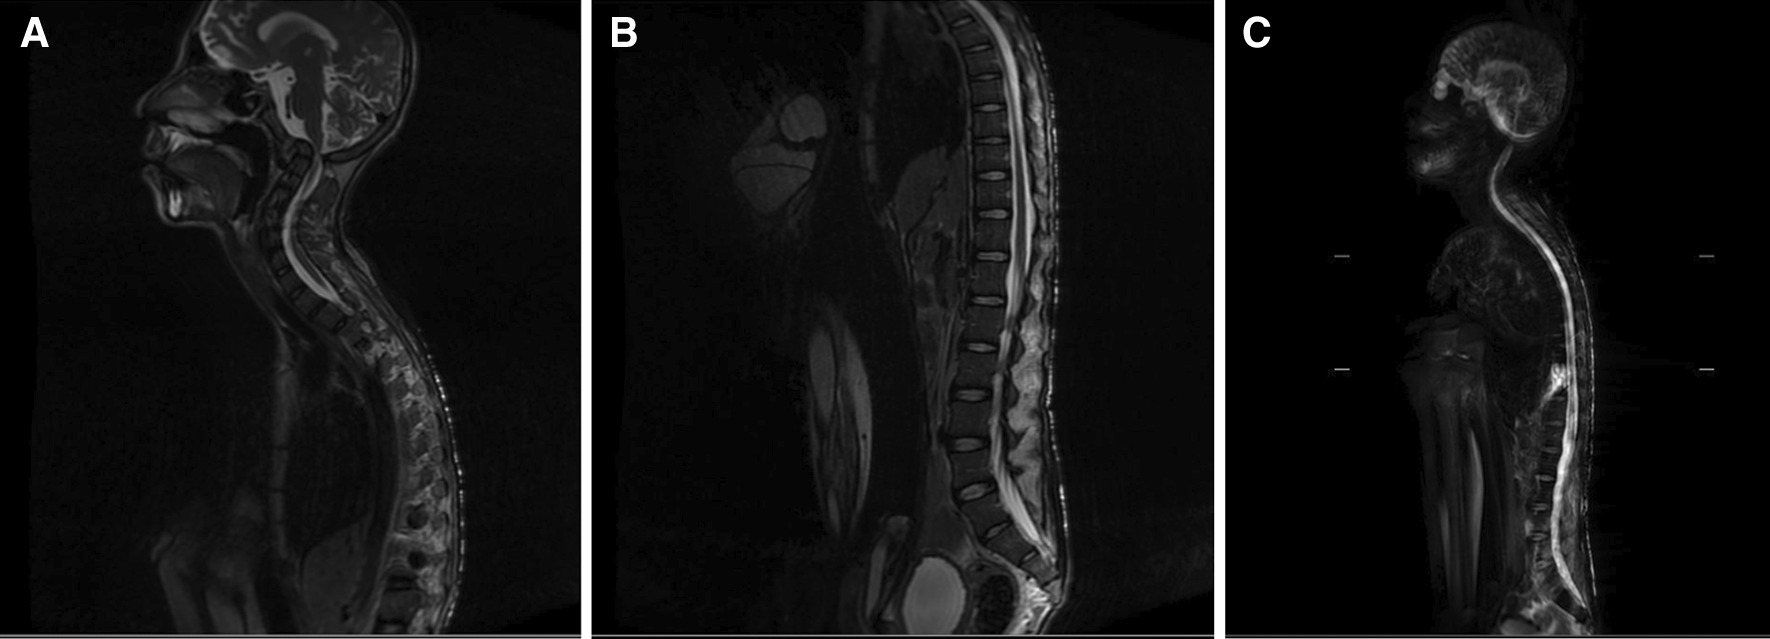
Sagittal MRI of cervical (A), thoracic (B), and whole spine (C) showing no remarkable cord compression or myelomalacia changes, with cervical hyperextension and thoracic kyphotic and scoliotic deformity
